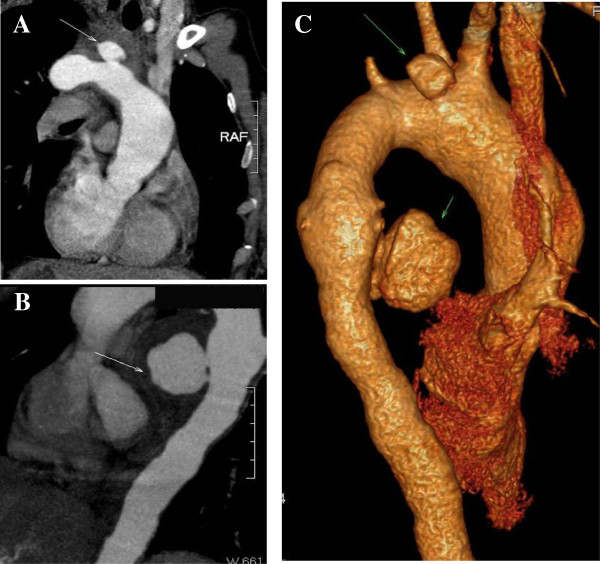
Computed tomography angiography images of preoperation. Its showing the aortic arch aneurysm (A) and descending aorta aneurysm (B). A 3-dimensional aortic image (C) was constructed.
